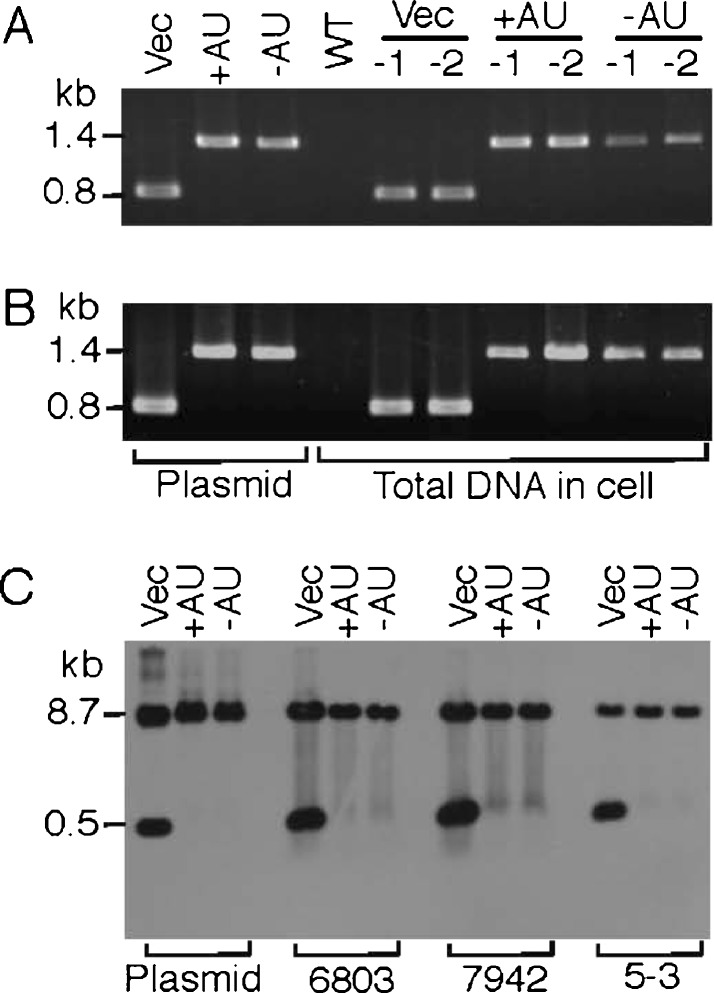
PCR analysis of recombinant *S*. *elongatus* PCC 7942 (a) and *Lymnothrix*/*Pseudanabaena* sp. ABRG5-3 (b) containing GFP expression vectors. Per strain, 1 μg of total DNA was analyzed by the same procedure as described in Fig. 2a. c Southern blot analysis of each expression vector’s copy number in PCC 7942 and ABRG5-3 transconjugants. The DNA of pVZ321 (Vec), pAM500 (+AU), and pAM461c (−AU) (*left block*, 0.0133 pmol) digested with the restriction enzymes *Hin*dIII and *Xho*I was subjected to hybridization with a specific probe as described in Fig. 2b for the concentration marker which was used for the trial calculation of plasmid copy numbers in the cells (Table 2). Total DNA (20 μg) was isolated from the respective transconjugants of PCC 6803, PCC 7942, and ABRG5-3, digested by the restriction enzymes, and also subjected to hybridization with the specific probe. Of note is that the exposure time for the X-ray film was approximately 1.5 times longer than that of Fig. 2b
